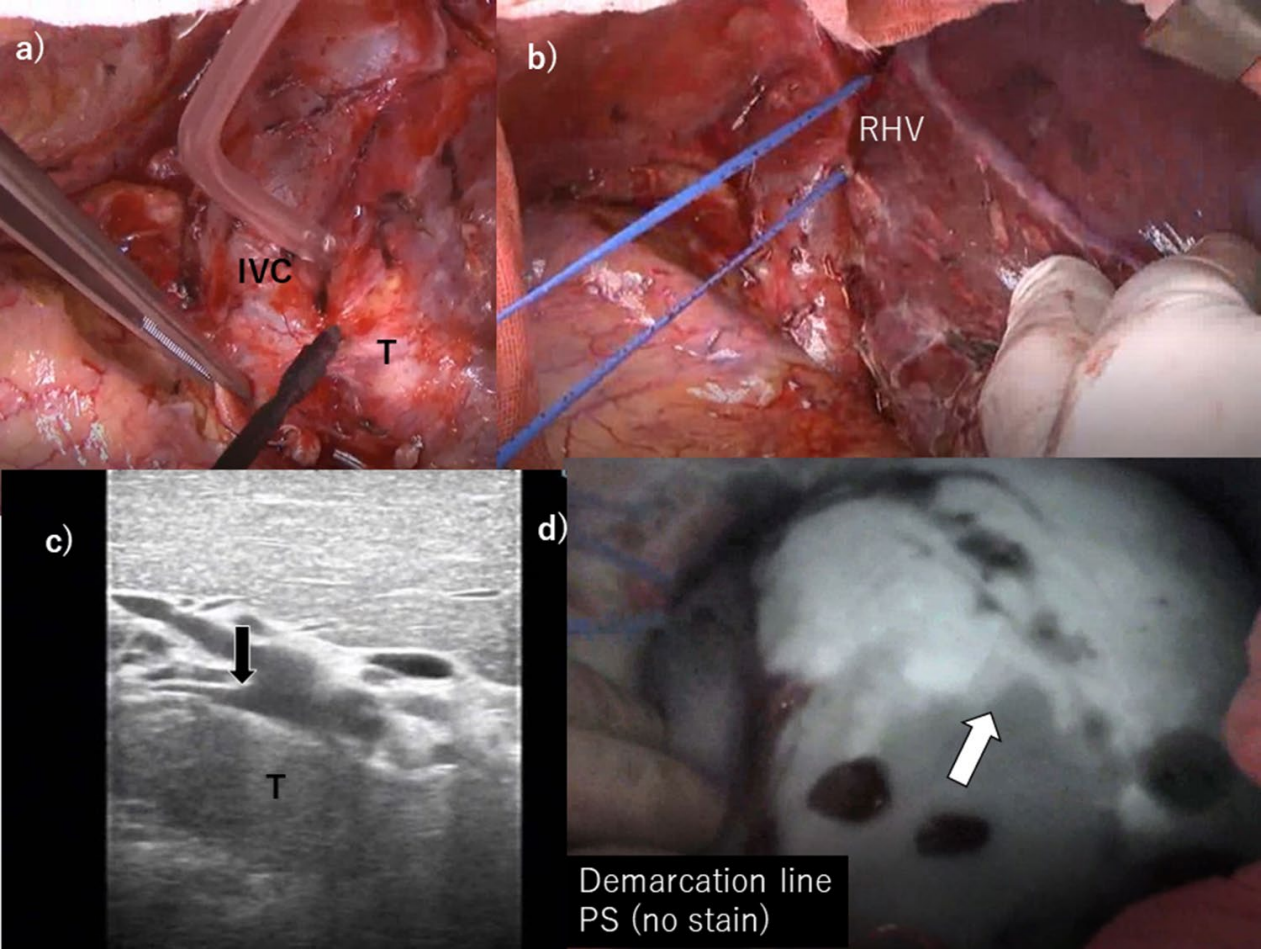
The intraoperative findings. a After mobilization of the right liver and cutting short hepatic veins, HCC in the segment 1 was gradually confirmed and adjacent to inferior vena cava (IVC) but not infiltration. b. The right hepatic vein (RHV) was secured and taped. c The intraoperative sonography showed the tumor (T) stenosis of the posterior portal pedicle and, however, it was possible to encircle the root of pedicle (black arrow). d After clamping the posterior portal pedicle, demarcation line of the posterior sector (PS) detected by the ICG photodynamic eye imaging (white arrow) was confirmed. Hepatic transection was started from this line
